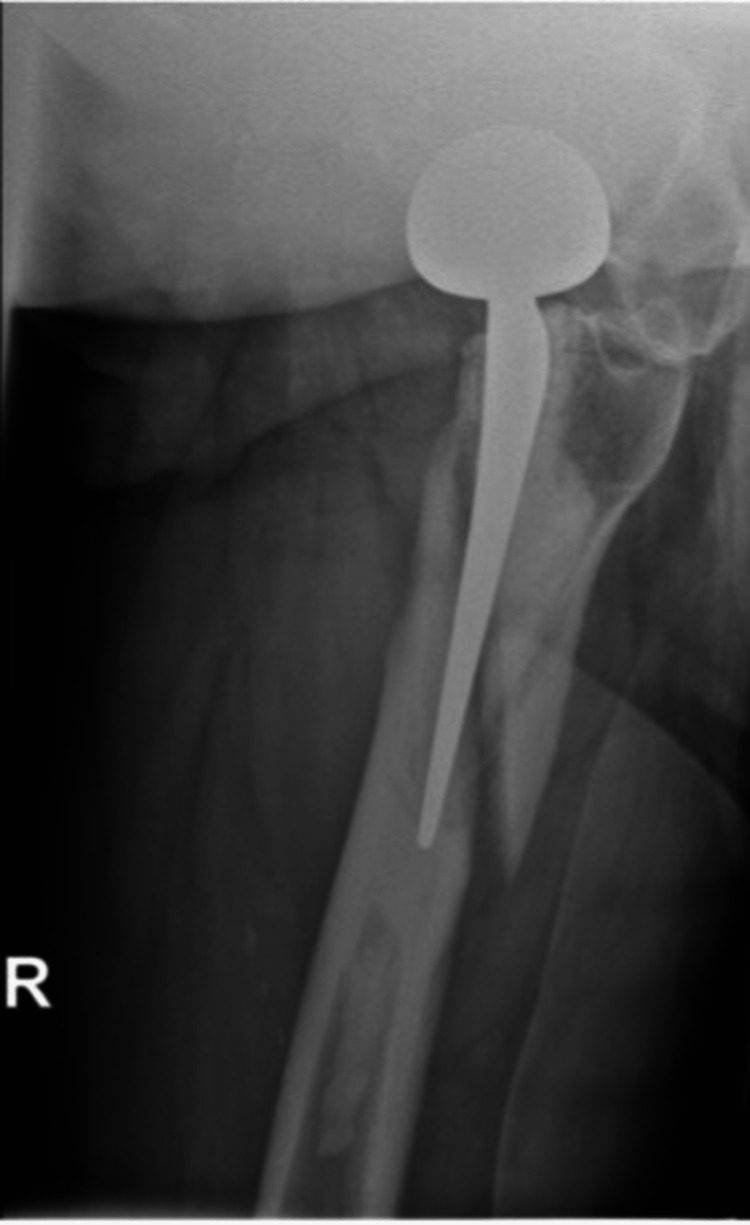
Case A, X-hip-axial pre-OR X-ray showing a periprosthetic femoral fracture of the right femur, Vancouver B2 type of fracture
